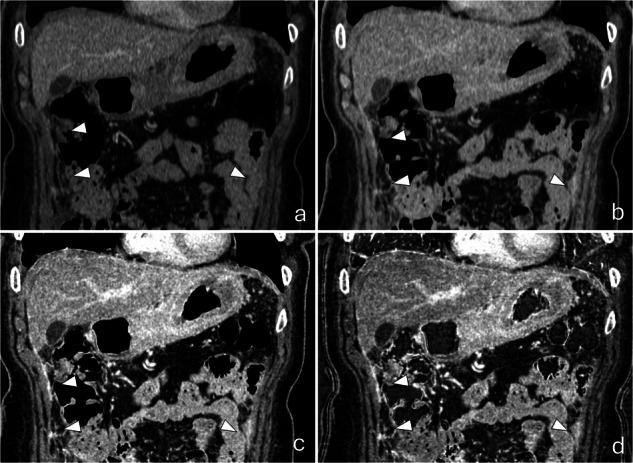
Example of DECT reconstruction advantages. Portal phase (a), delayed virtual 120-kV (b), delayed VMI-40-keV (c), iodine maps (d). Patients showed PM in regions 1, 8, and 4 (arrowheads). There were differences in readers’ calculation of PCI when employing DECT: the mean PCI was 1.3 *versus* 2.9 for region 1, 0.6 *versus* 1.8 for region 8, and 1.0 *versus* 1.7. for region 4. CT, Computed tomography; DECT, Dual-energy CT; PCI, Peritoneal cancer index; PM, Peritoneal metastases
